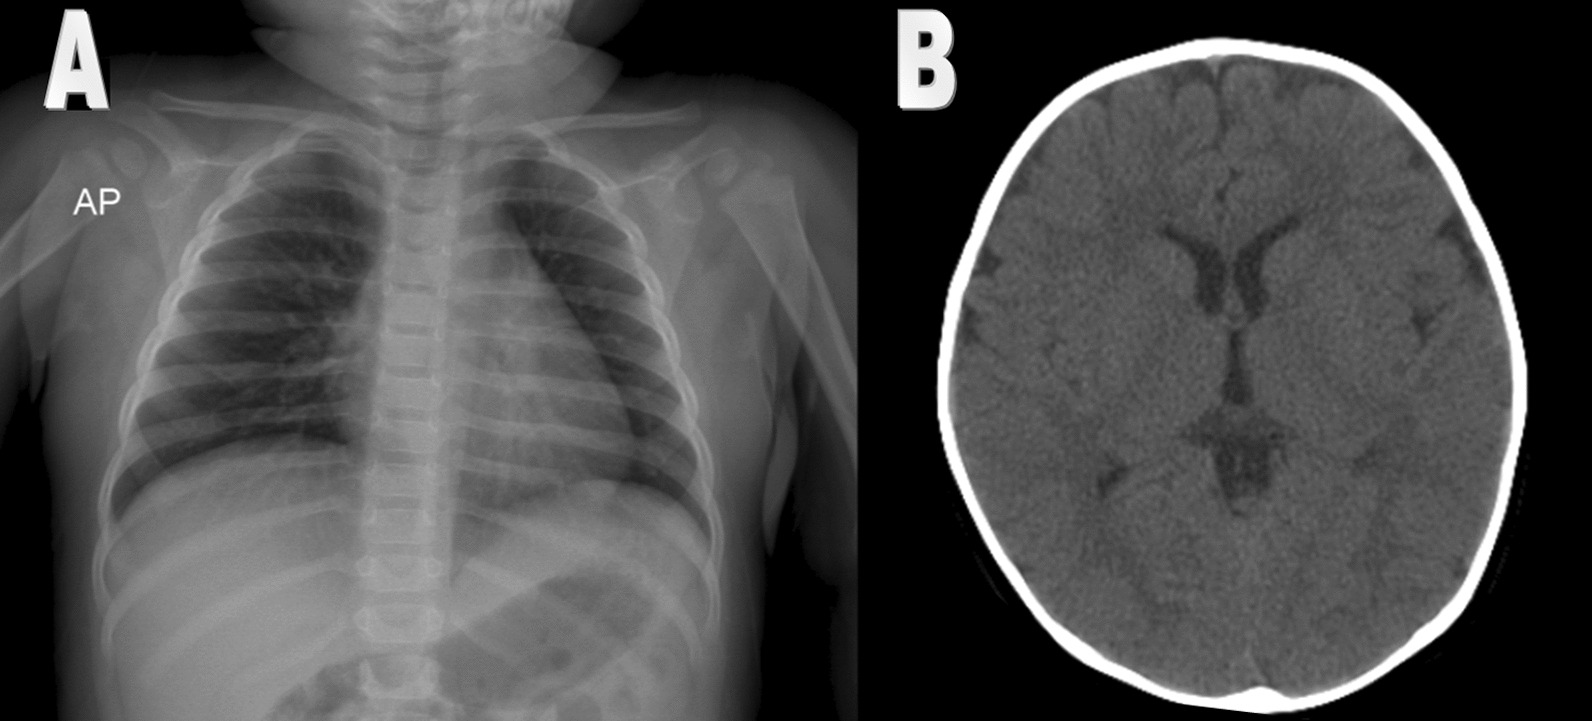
In case 3 chest X ray (A) revealed reticular changes and Brain CT scan (B) was normal
